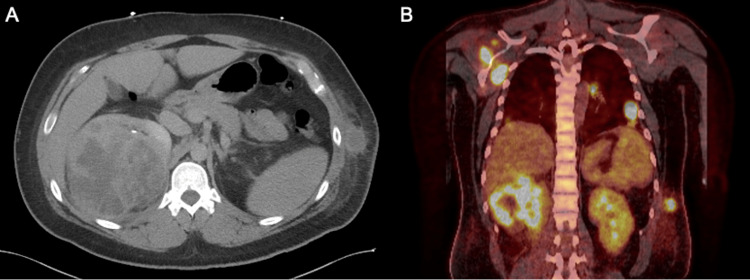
Case 1 - imaging of the right renal mass at initial presentation. (A) Axial computed tomography of the chest, abdomen, and pelvis demonstrates a heterogeneous, complex mass at the upper pole of the right kidney. (B) Positron emission tomography/computed tomography shows intense hypermetabolic activity of the right renal mass, with additional FDG-avid lesions in the lungs, scapula, and left lateral chest wall. FDG: fluorodeoxyglucose
